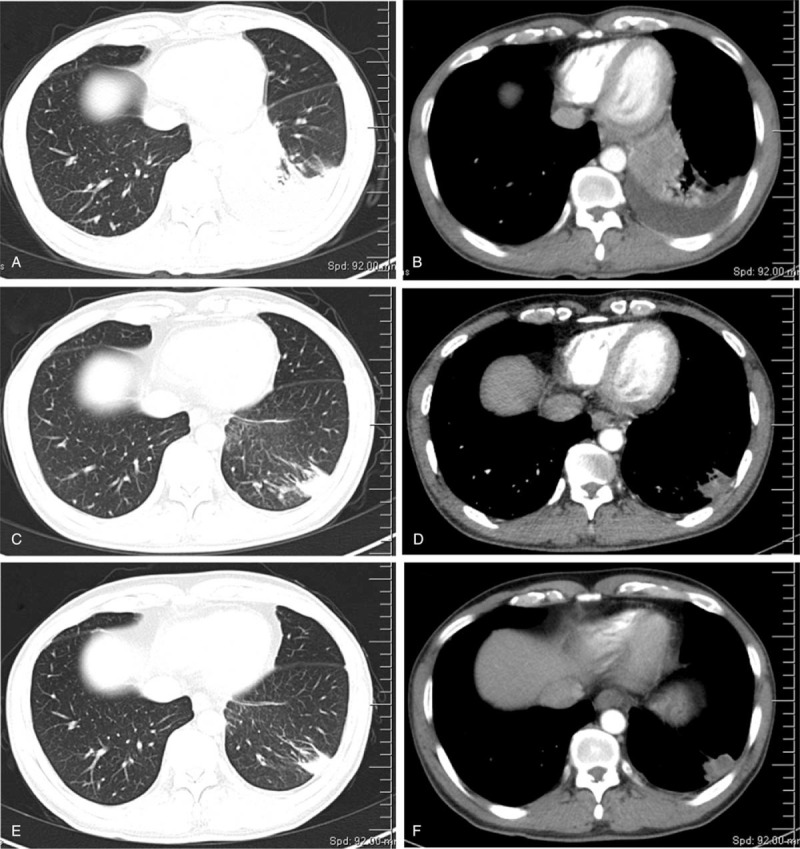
Chest CT scans of the patient before and after treatment. A-B: First chest CT scan taken on February 13, 2020, note the lesion and pleural effusion in the left lung; C-D: Reexamination of chest CT scan taken on April 20, 2020 after two cycles of treatment; E-F: Reexamination of chest CT taken on June 3, 2020 after four cycles of treatment.
